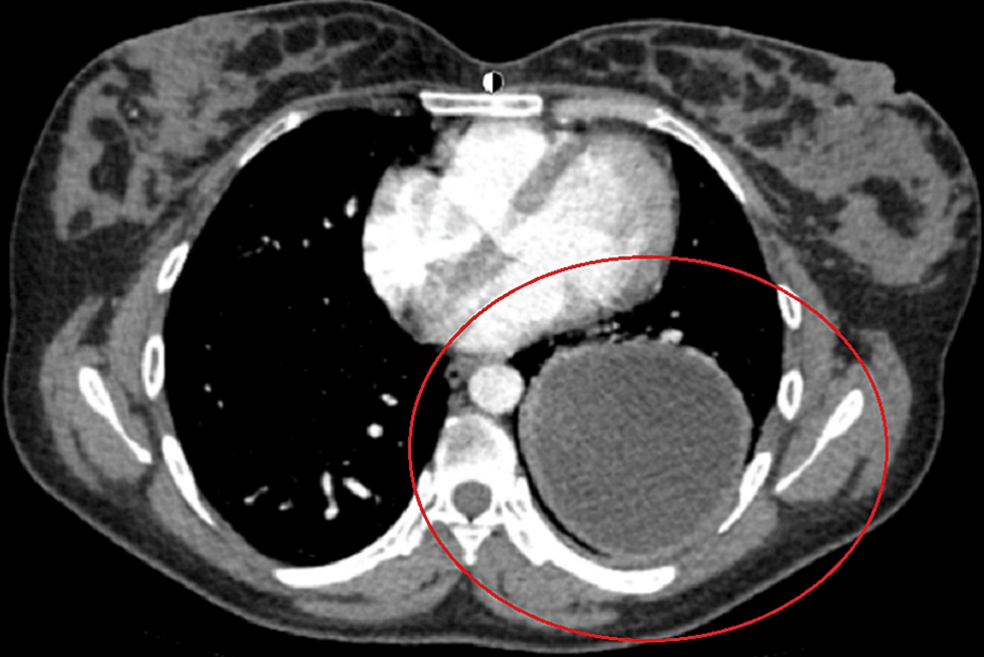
Axial CTA with a large fluid density and well-circumscribed, homogenous margins in the right posterior lung. CTA - computed tomography angiography
